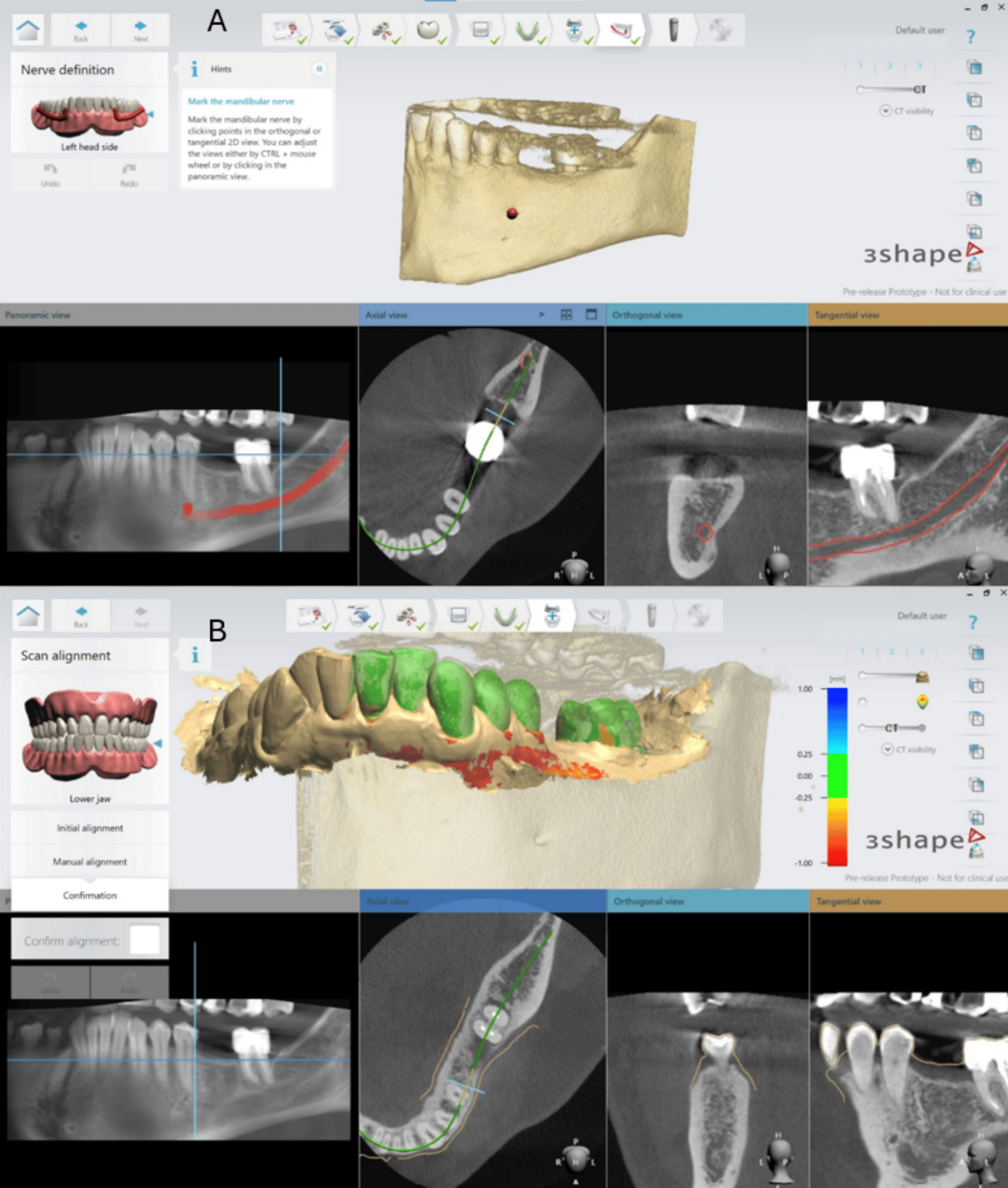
CBCT scan processing: panoramic curve adjustment, alignment of intraoral and CBCT scans (A), and marking of the mandibular nerve (B) CBCT: cone beam computed tomography
